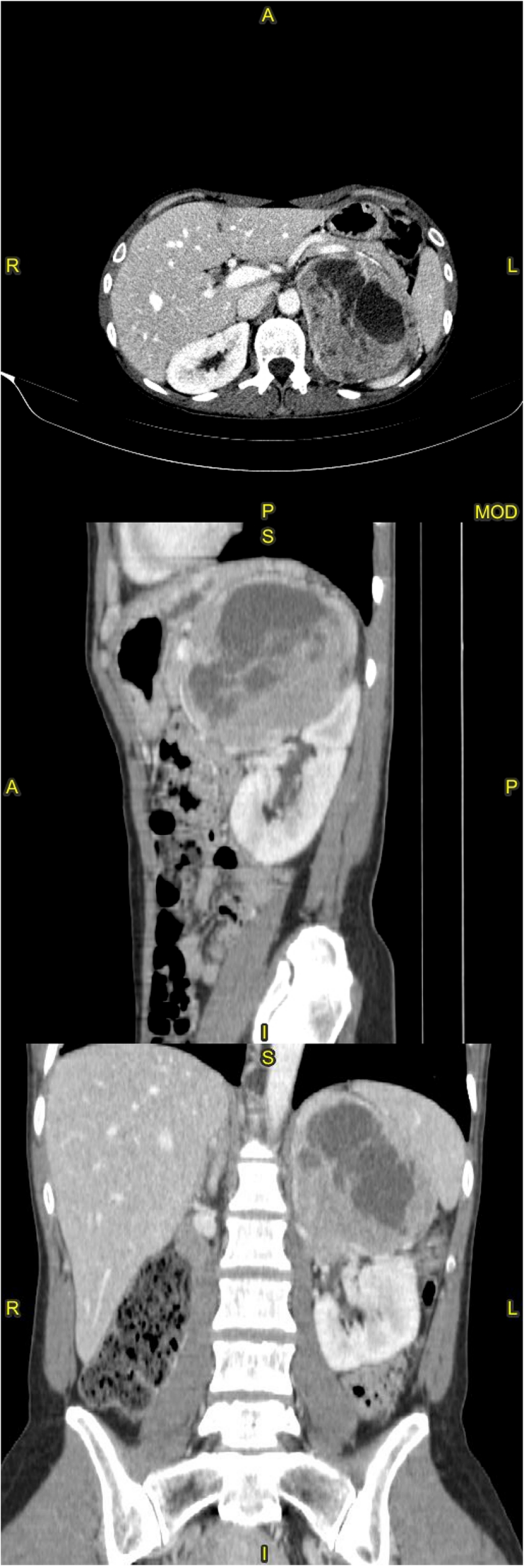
Multiplanar reconstruction of a contrast-enhanced abdominal computed tomography scan. The left adrenal mass has a maximum diameter of 11.2 cm, and is seen displacing the gastric fundus antero-superiorly and the spleen laterally; cleavage from the kidney is unclear around the pelvis in the coronal image, suggesting cancerous infiltration
